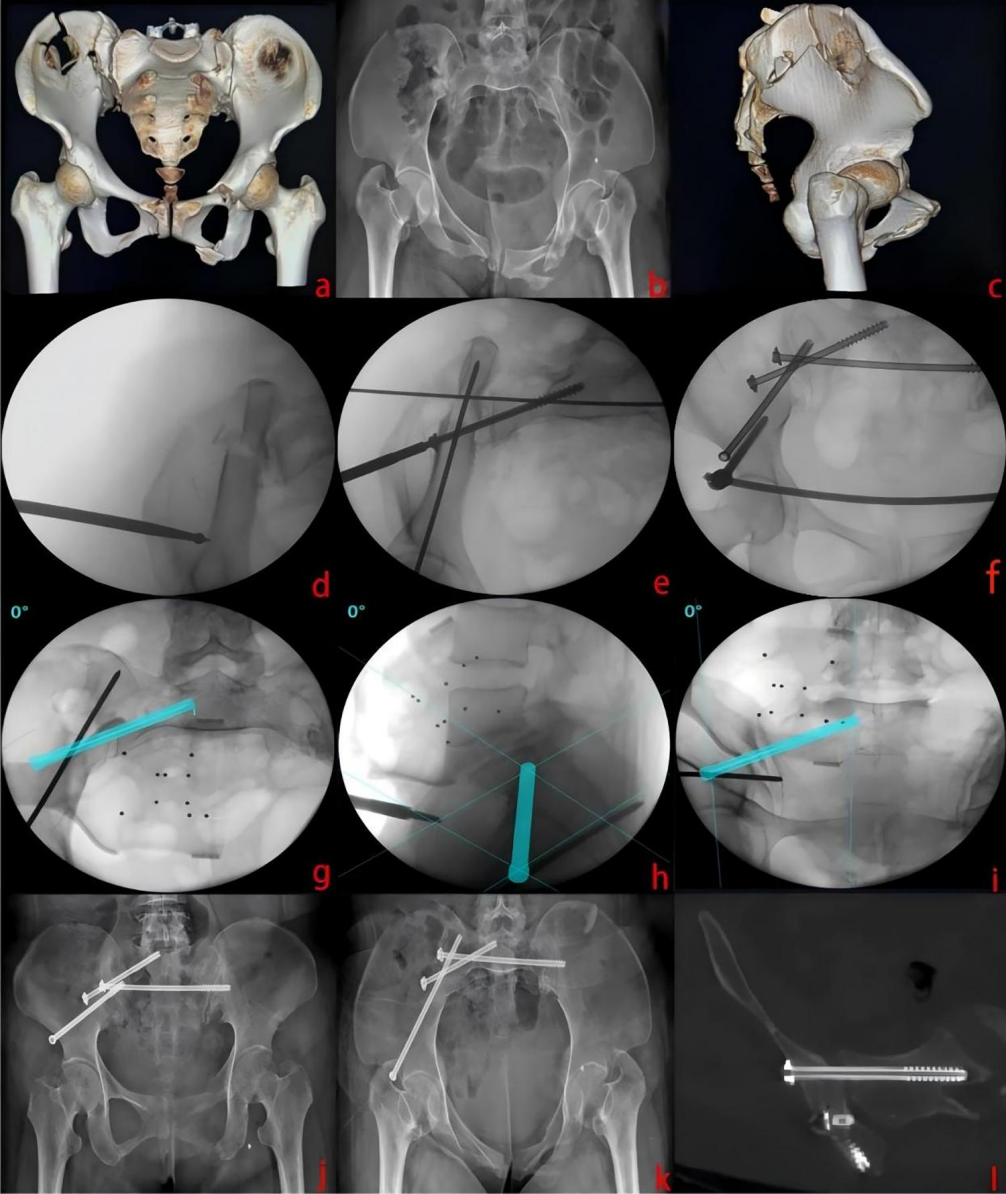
Pre-operative CT and x-ray images of a 44-year-old female patient with a Day type II CFDP (a–c). Through a 1-cm incision, fracture reduction was achieved with an apex cone on the iliac outer table, followed by fixation with a guide pin and subsequent placement of modified SI, LC-II, and S2 screws, with anterior stability provided by INFIX (d–f). Intraoperative modified sacroiliac screw planning images (g–i). One-year follow-up imaging confirmed anatomical reduction, good fracture healing, and intact implants (j–l).
